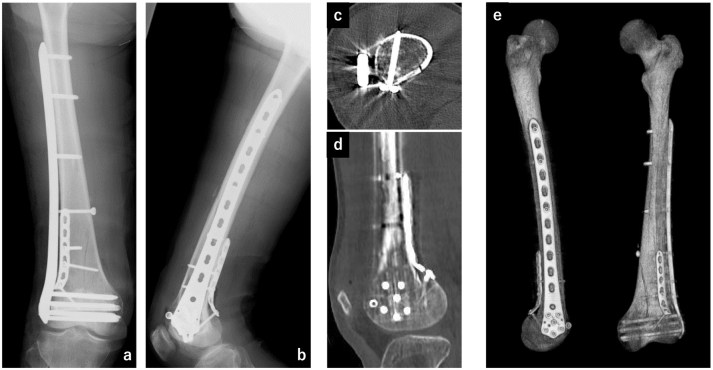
Postoperative plain X-ray and CT scan of the distal femur. a: A-P plain radiograph, b: lateral plain radiograph, c: CT axial slice image showing the perpendicular plate application. d: A buttress plate was applied at the ideal position of the posterior aspect of the distal femur lateral condyle. e: Postoperative 3DCT picture.
